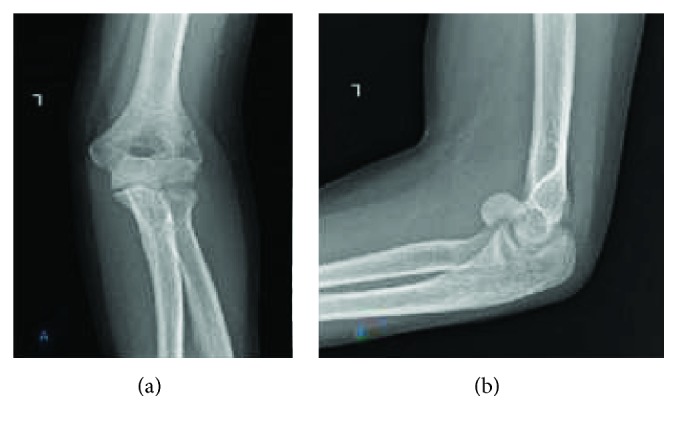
Preoperative left-elbow plain X-rays. (a) Anteroposterior and (b) lateral.
